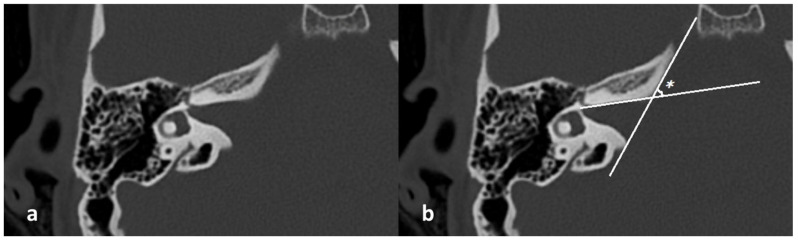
Measurement of the lateral angle (LA) on axial temporal bone CT. (a) Axial section at the level of the lateral semicircular canal and the geniculate ganglion. (b) Tangent lines (*) to the IAM inlet and anterior wall form the LA (°).
